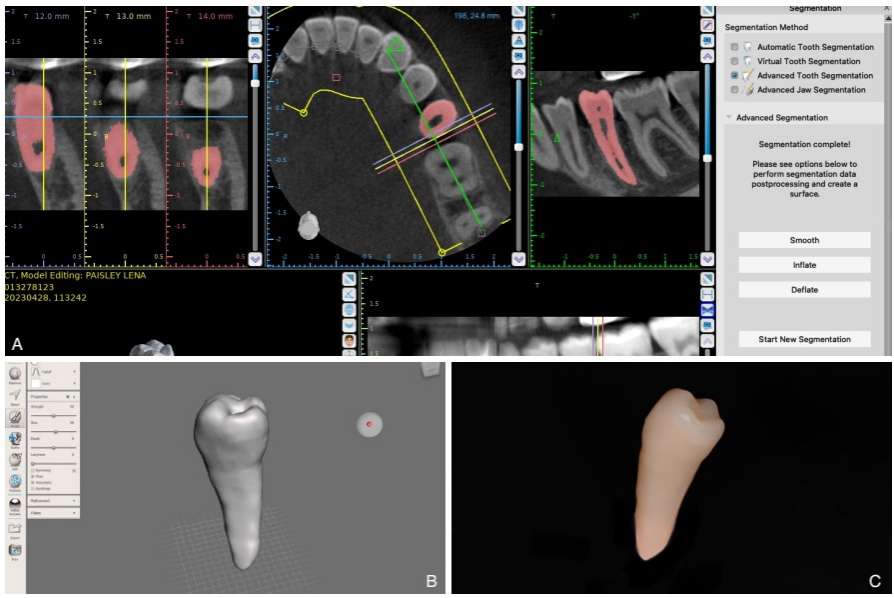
Digital planning and 3D-printed CARP models of the donor tooth. A) Extraction of the dental volume from CBCT in STL format and segmentation of the donor tooth #35 (in red) on Blue Sky Plan®. B) Thesmoothing of the volume obtained by additions on Meshmixer. C) Machined dental replica of tooth 35.
